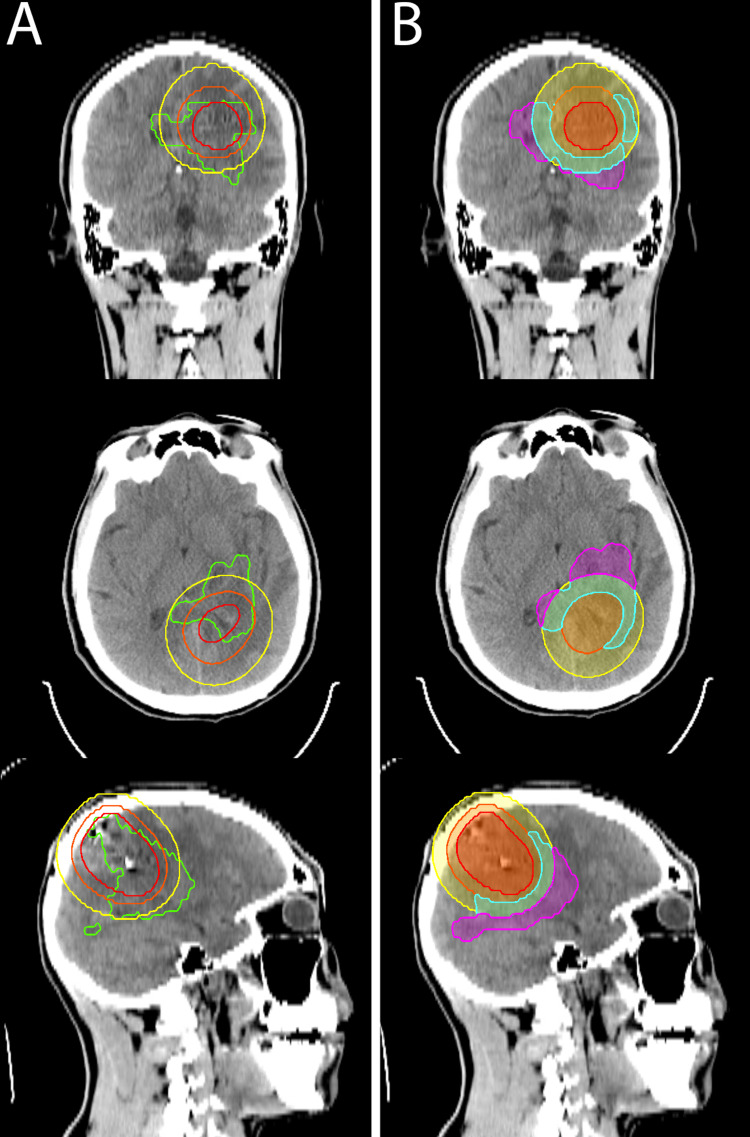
Development of post GammaTile contours and planning target volumes. Figure 2A (Left) shows the GTV (green line) on coronal, axial and sagittal CT images against the 17.5 Gy (yellow line), 35 Gy (orange line) and 60 Gy (red line) isodose lines from GammaTile delivery. Figure 2B (right) shows the ultimate planning volume consisting of two PTVs. PTV-1 (shaded magenta) overlaps with tissue that received less than 17.5 Gy from GammaTile (no shade) and PTV-2 (light blue shade) overlaps with tissue that received 17.5 Gy to up to 35 Gy (shaded yellow). The volume that received 35 Gy or more (shaded orange and red) were not included in the PTVs. Volumes and contours displayed in Velocity™ (Varian Medical Systems, Palo Alto, California).
